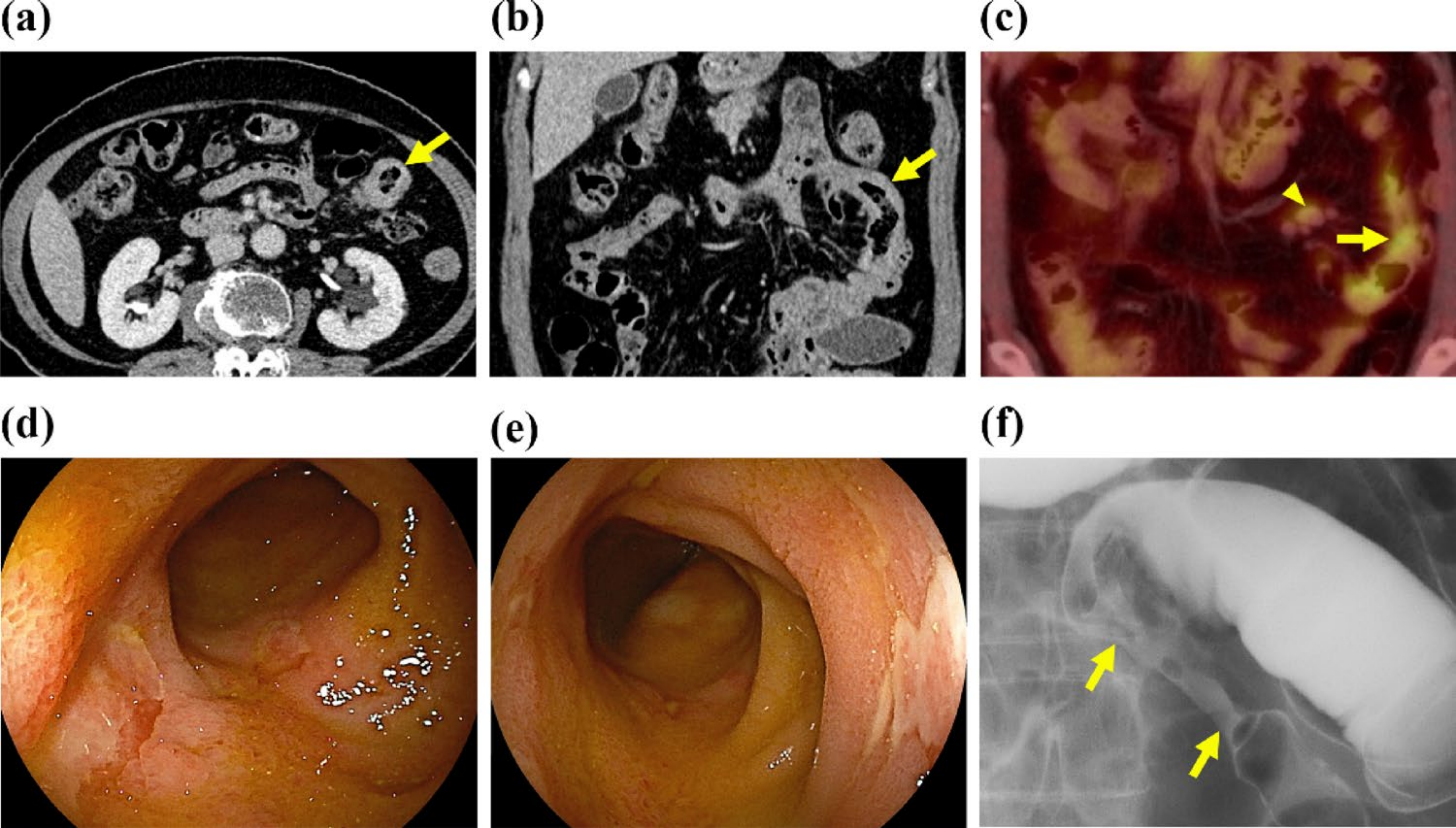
Radiological and endoscopic images of the small intestinal lesion in Case 1. a, b Axial and coronal enhanced computed tomography images showing thickening of the proximal jejunal wall with luminal narrowing, upstream dilatation (yellow arrows). c FDG PET/CT images showing intense FDG uptake in both the thickened jejunal segment (SUVmean = 10.0; SUVmax = 11.6, yellow arrow) and the enlarged lymph nodes (SUVmean = 7.4; SUVmax = 8.8, yellow arrowheads). d, e Antegrade double-balloon enteroscopy (EN-580T; Fujifilm, Tokyo, Japan) reveals multiple shallow ulcers in the ileum. f Contrast injection during enteroscopy delineates a mild 40-mm-long luminal stenosis (yellow arrows)
